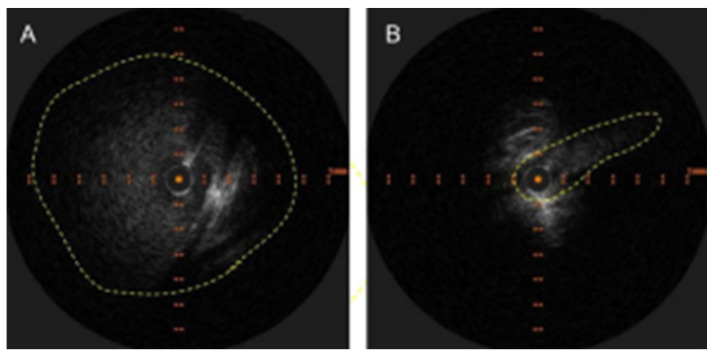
(A) Appearance of LBV on intravascular ultrasound with manual demarcation of the approximate border with dashed yellow lines. (B) Severe slit-like compression of LBV.
